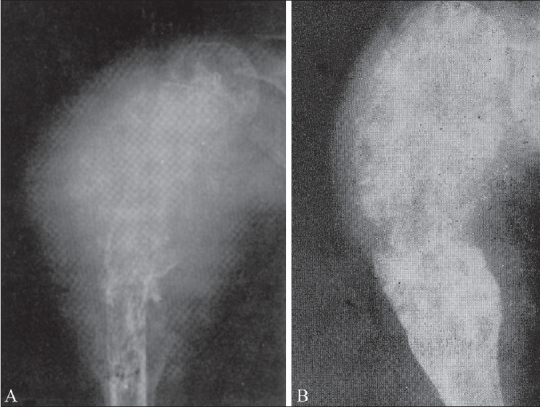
Ewing's sarcoma of the humeus. Pre-irradiation radiograph (A) shows a destructive lesion with a “honey-comb” appearance. The post-irradiation radiograph (B) shows a mottled appearance with sclerosis and residual areas of osteolysis
